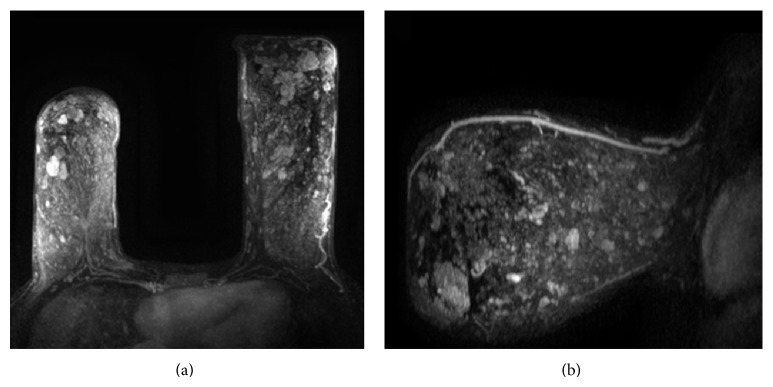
Imaging findings. Contrast enhanced T1 MRI demonstrates innumerable lobulated enhancing masses within both breasts as well as macromastia (a). Sagittal contrast enhanced T1 MRI of the left breast showing macromastia and innumerable lobulated enhancing masses (b).
